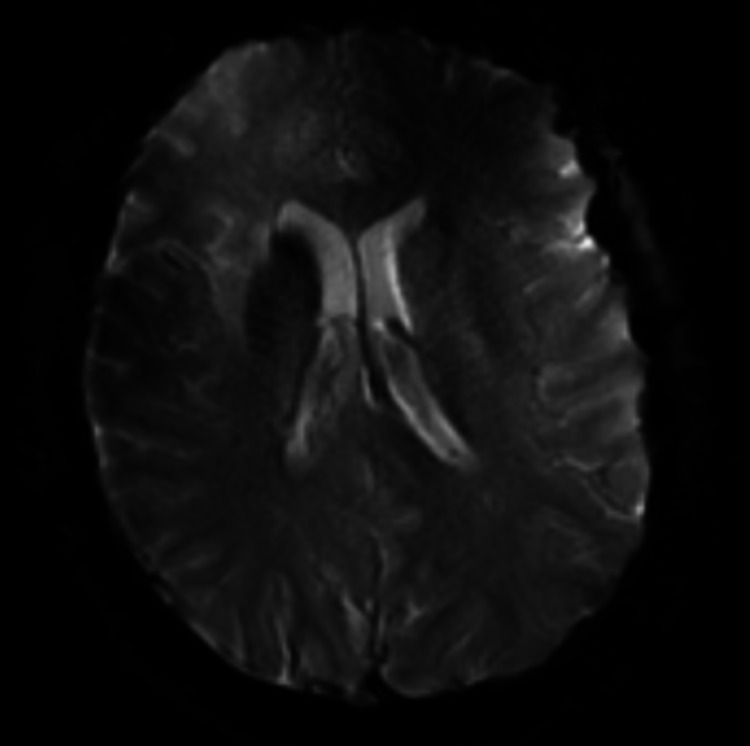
MRI gradient echo demonstrating hypointensity within the right basal ganglia reflecting calcification. MRI: magnetic resonance imaging.
